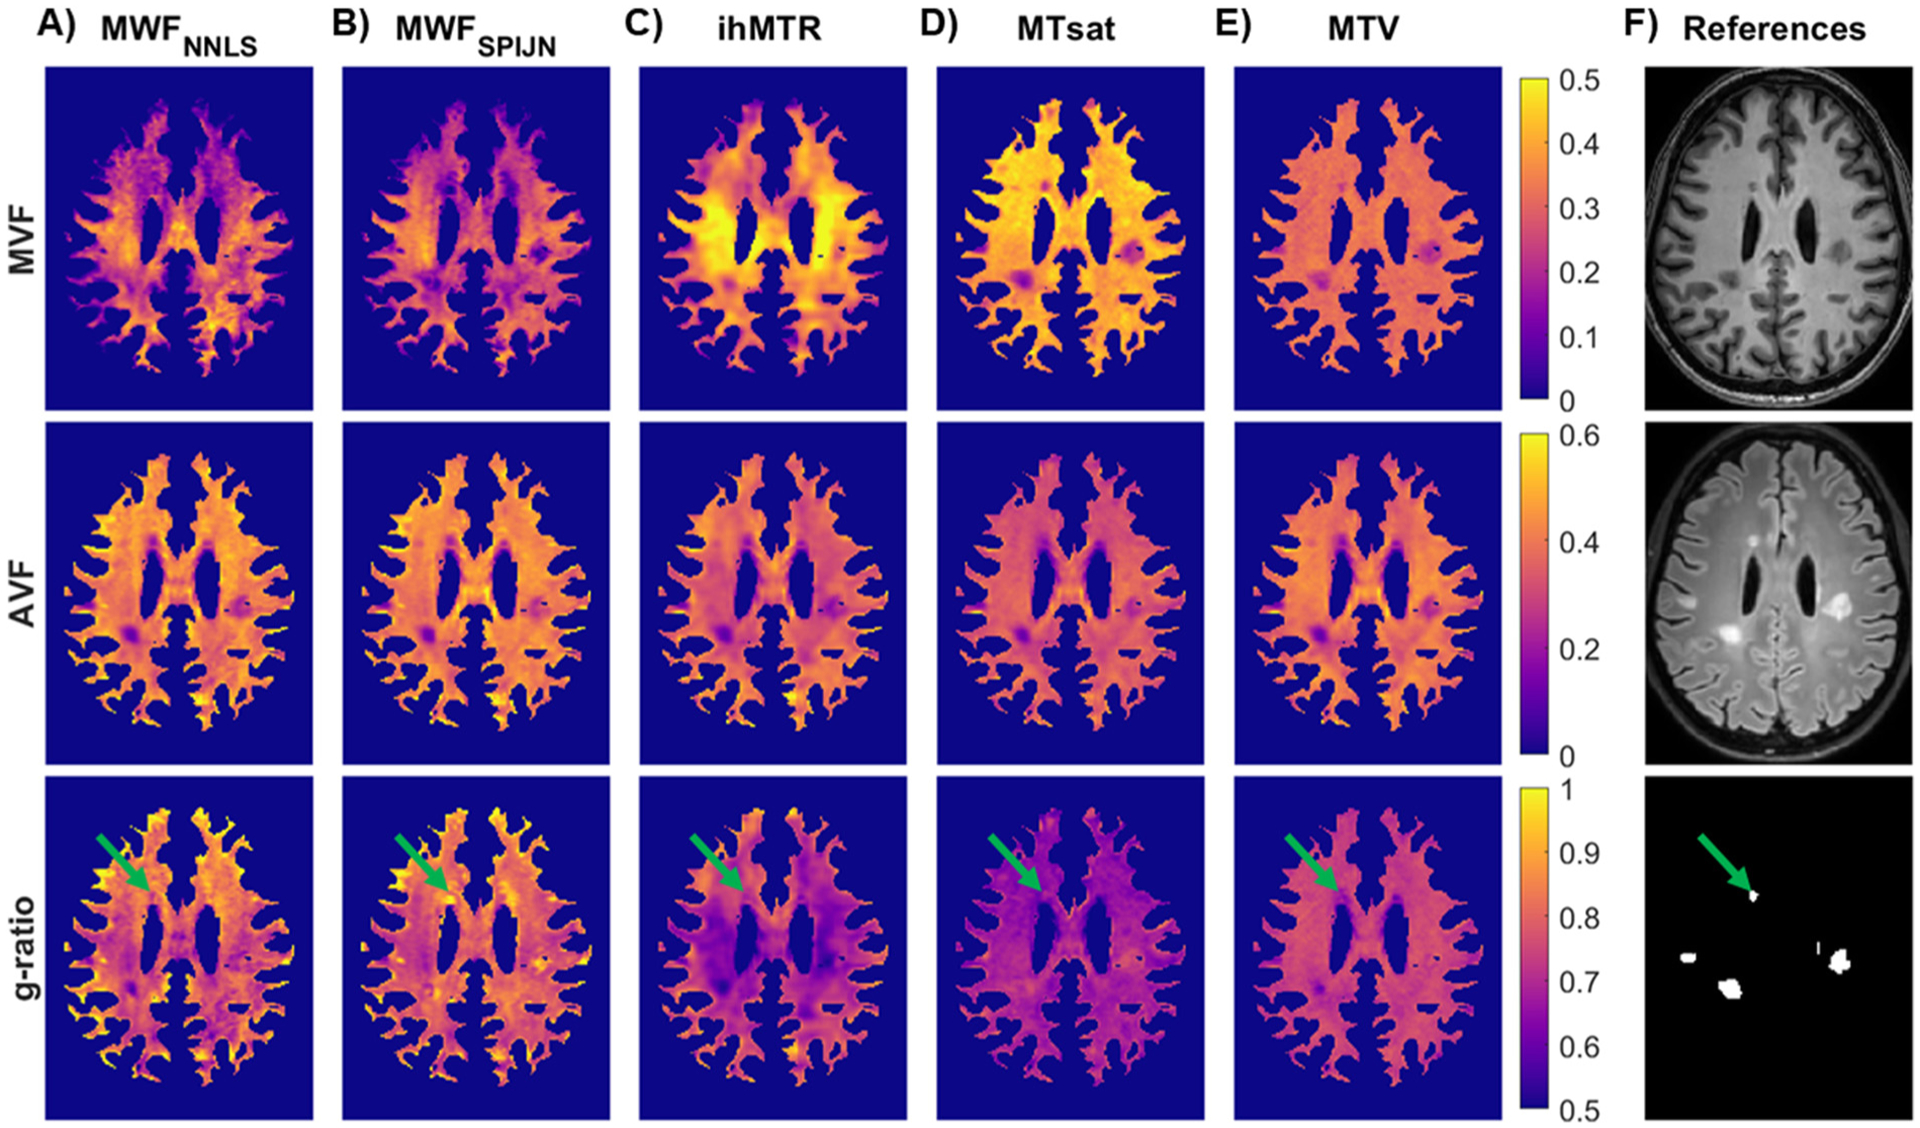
Representative slice of myelin volume fraction (MVF), axonal volume fraction (AVF), and g-ratio parameter maps from an MS patient calculated based on different myelin-sensitive measures. Myelin-sensitive measures include MWI using two different fitting techniques for the myelin water fraction, MWF_NNLS_ (A) and MWF_SPIJN_ (B), as well as ihMTR (C), MTsat (D), and MTV (E). As reference, the MPRAGE (top), FLAIR (middle), and lesion mask (bottom) are shown in the last column (F). Green arrows point to an MS lesion that shows differences in the g-ratio values between different g-ratio mapping methods.
